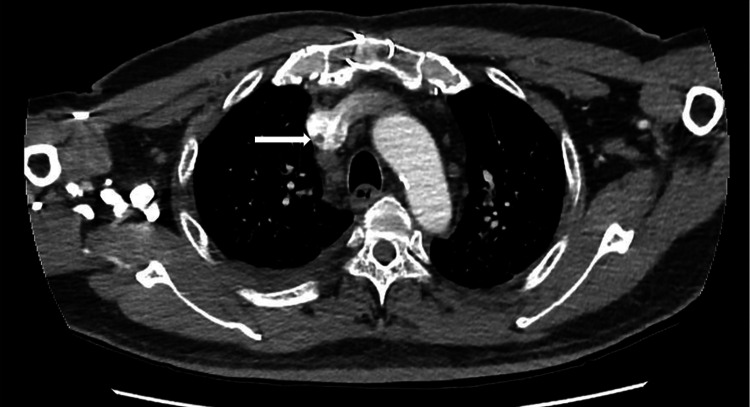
CT venogram demonstrating presence of venous fibrin sheath (after catheter removal). CT, computed tomography.
